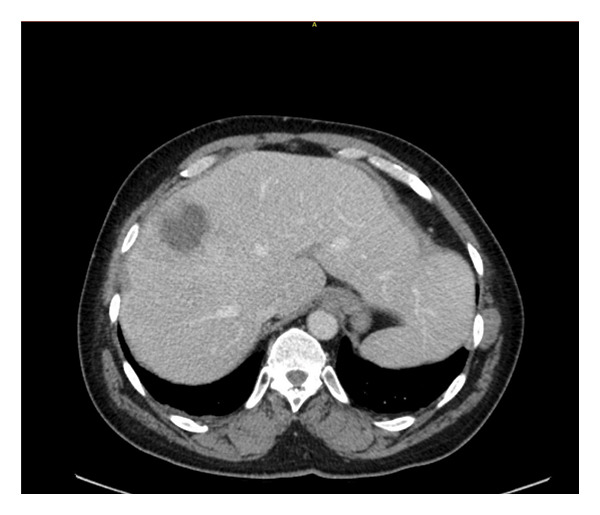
Cross‐sectional view of computed tomography (CT) demonstrating hepatic abscess (white arrow) in the right lobe.
